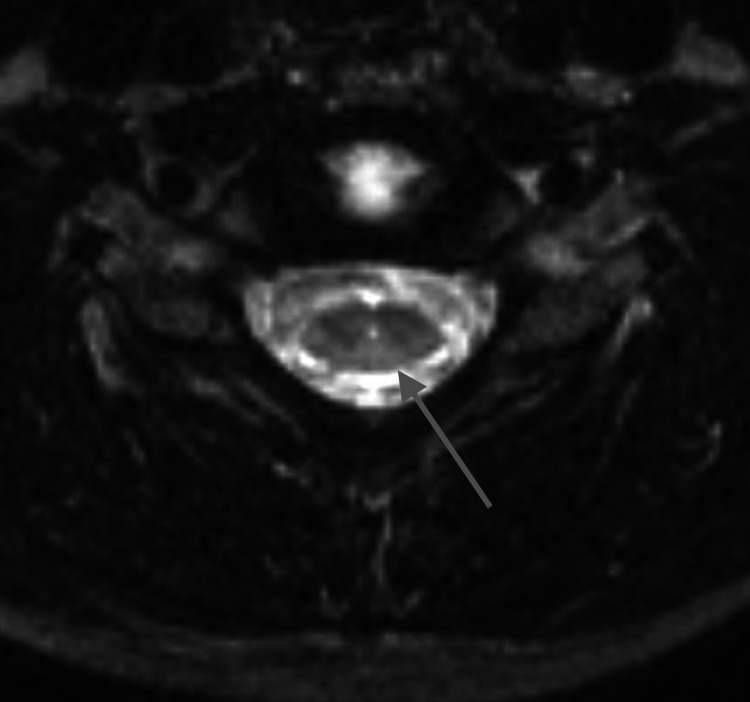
Axial T2-weighted image of the spine at the level of C6-7 at five years of age shows a slit-like syrinx (gray arrow)
